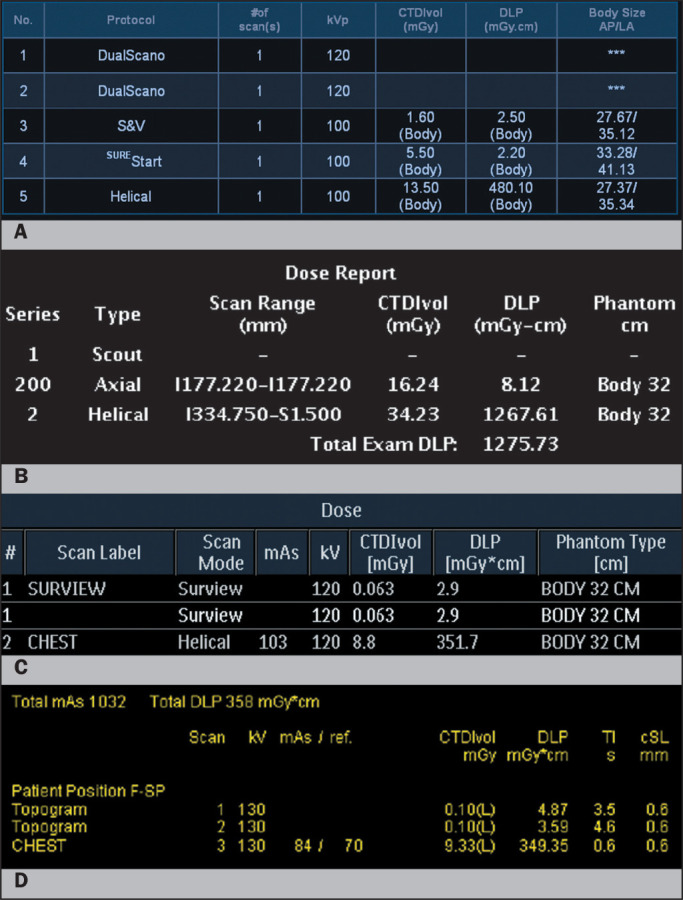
Dose information pages from four major CT vendors (A: Canon; B: GE; C:
